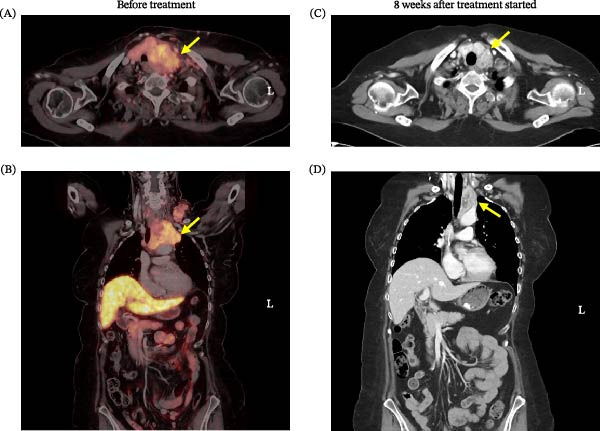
Radiological examinations of Case 2. ^68^Ga‐DOTATOC‐PET/CT scans (A,B) prior to the start of treatment with TKI reveal large tumors on the left side of the thyroid, along with extensive lymph node metastases in ipsilateral regions 2–4. A CT scan performed 8 weeks after the start of treatment is shown, with an overall reduction in size of the tumors indicating a partial radiological response. Transverse (A,C) and coronal (B,D) sections are presented, with “L” indicating the left side. Yellow arrows indicate the thyroid tumor.
